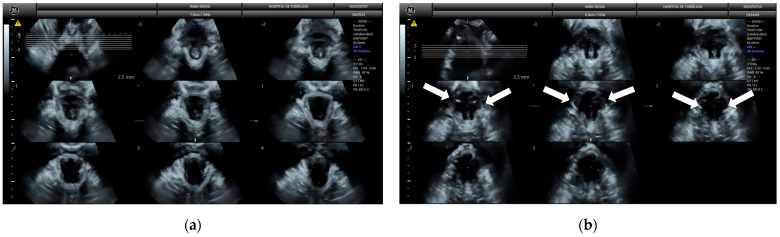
Tomographic ultrasound imaging (TUI) of normal levator ani muscle insertion (a) and complete bilateral avulsion of the levator ani muscle (b), demonstrated by absence of visible muscle attachment at the pubic bone across the central slices (white arrows).
